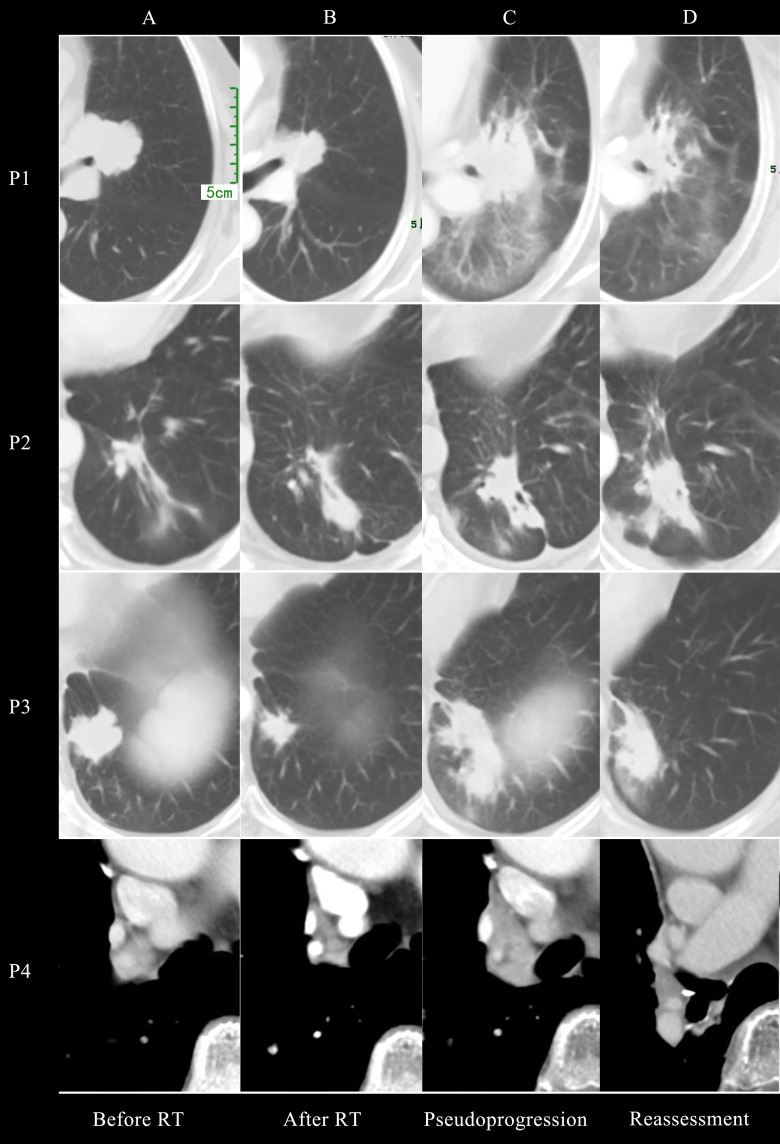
Chest CT imaging of all 4 patients before radiotherapy (A), after radiotherapy (B), at pseudoprogression (C) and first reassessment after pseudoprogression (D).
